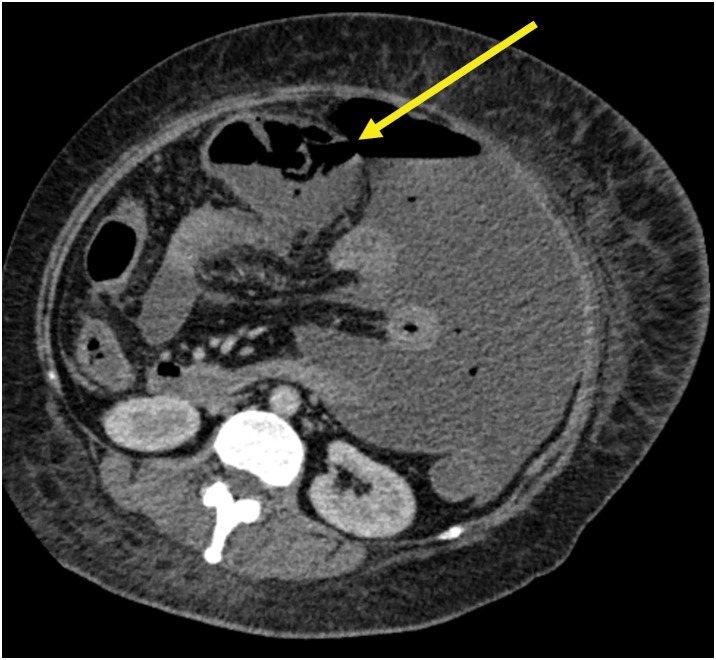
Abdominal CT on hospital day 8. Perforation of the transverse colon wall (yellow arrow), accompanied by massive ascites and free air, was observed.
